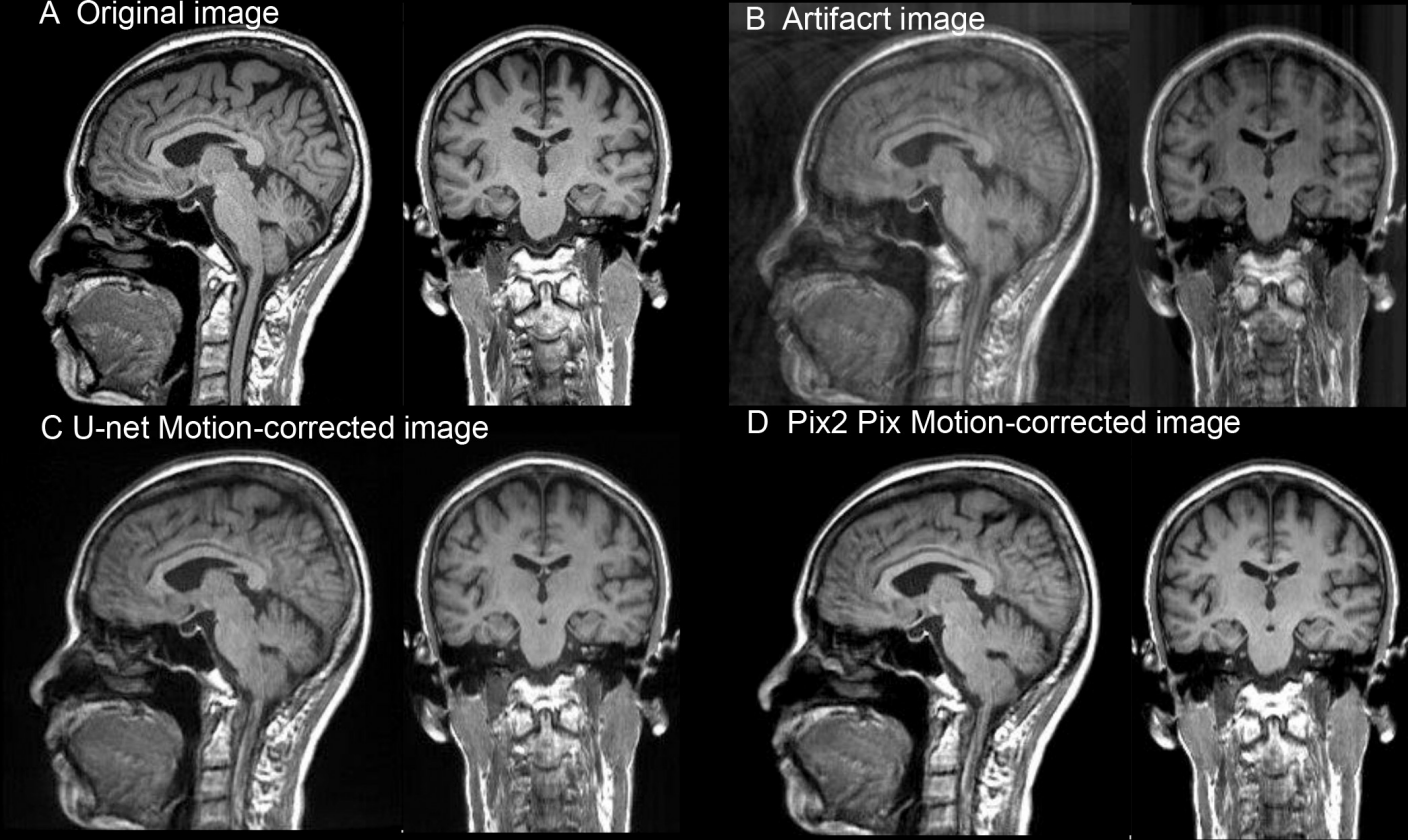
Examples of the original image, artifact image, and motion-corrected image. (A) The original T1-weighted, magnetization-prepared rapid gradient-echo sequence (T1-MPRAGE) images, (B) artifact images created by manipulating the k-space of T1-MPRAGE, (C) motion-corrected images by the U-Net network from the artifact images and (D) motion-corrected images by the Pix2Pix network from the artifact images, are shown, respectively. The rotation angle and k-space interval of the artifact images were 2°_20 pixels.
